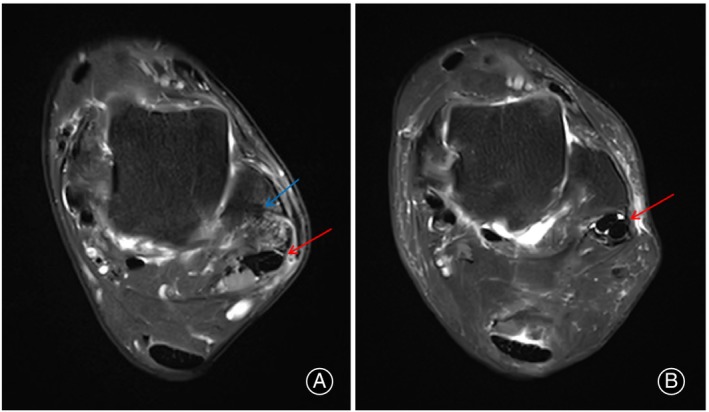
Postoperative MRI. (A) Position of suture anchor (blue arrow) and knot of wire (red arrow); (B) Complete continuity of SPR (red arrow).
